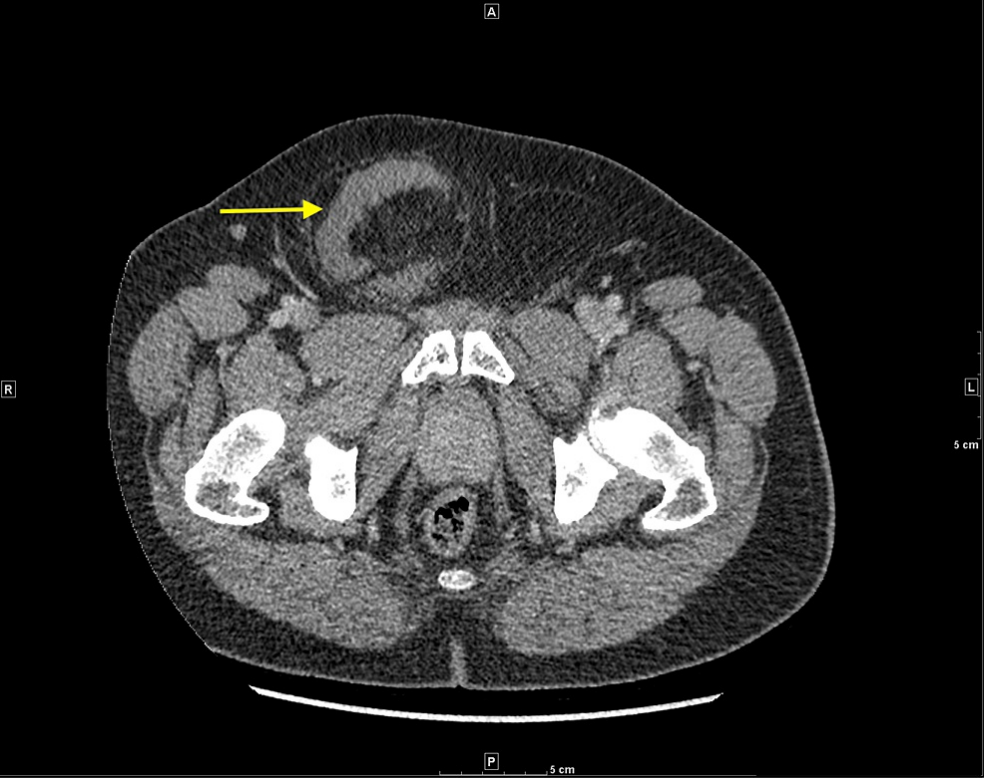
Axial CT image of small bowel segment traversing abdominal wall defect into the hernia sac (yellow arrow).
